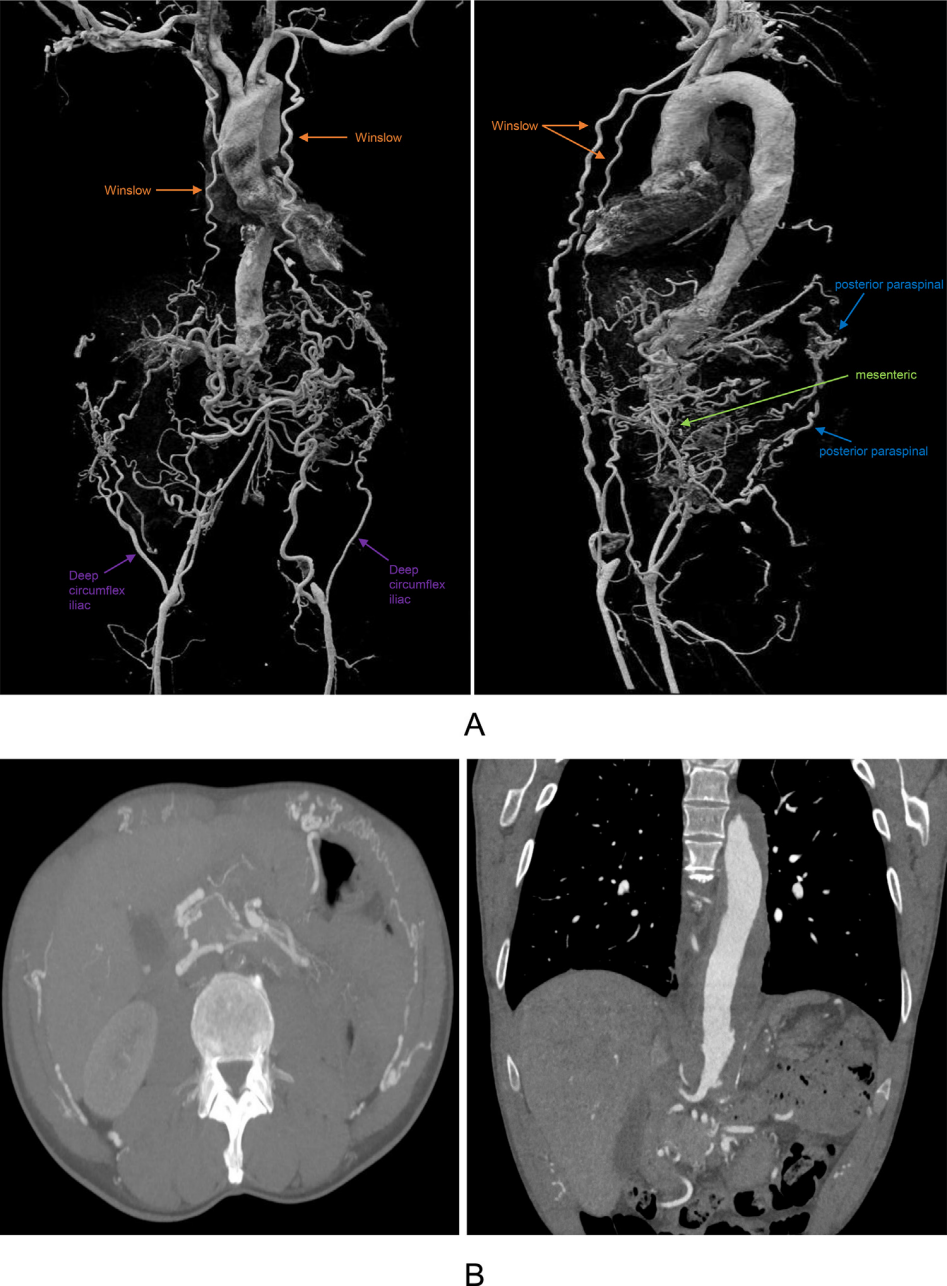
(A) Cinematic VRT demonstrating aortoiliac occlusion with bilateral Winslow pathway and additional collateral circuits (posterior paraspinal, mesenteric, deep circumflex iliac) and (B) Multiplanar CTA demonstrating aortoiliac occlusion with bilateral Winslow pathway and additional collateral circuits (posterior paraspinal, mesenteric, deep circumflex iliac).
